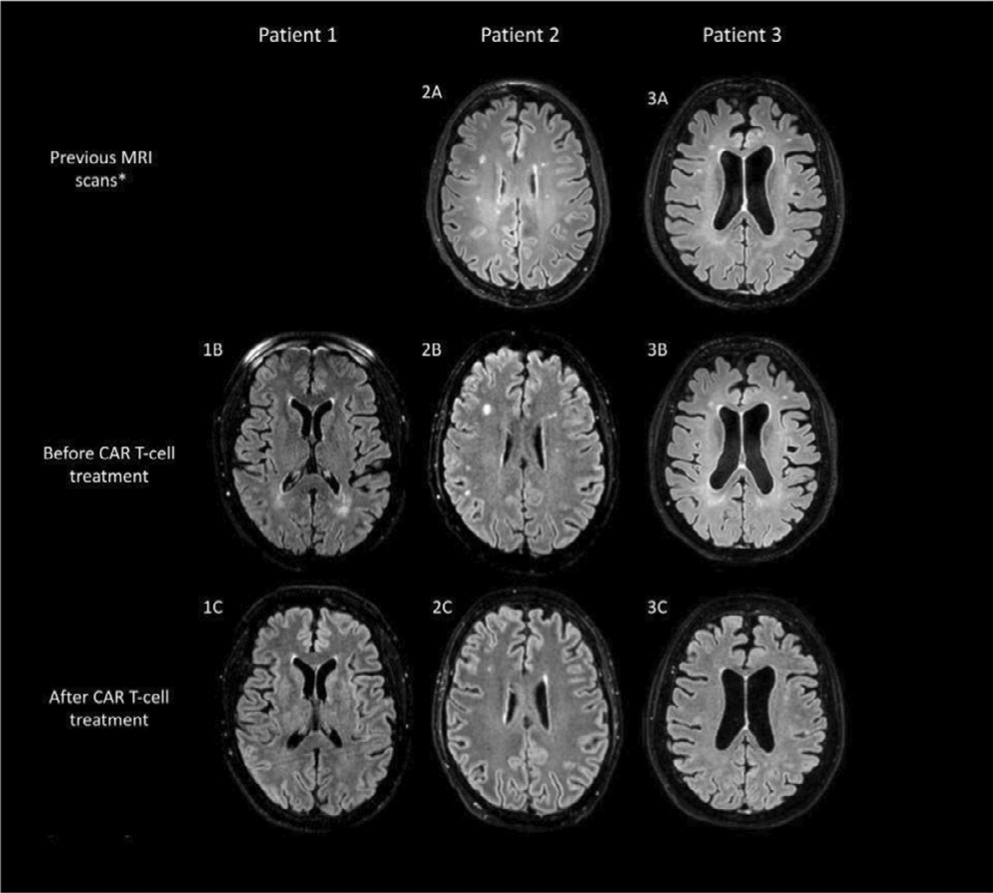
FIGURE 1 Axial MRI FLAIR of patients 1 to 3. “A” and “B” show subcortical white matter hyperintensities (WMH) in scans prior to CAR T‐cell treatment. “C” shows the disappeareance or decrease in size of the same WMHs, after CAR T‐cell infusion.
